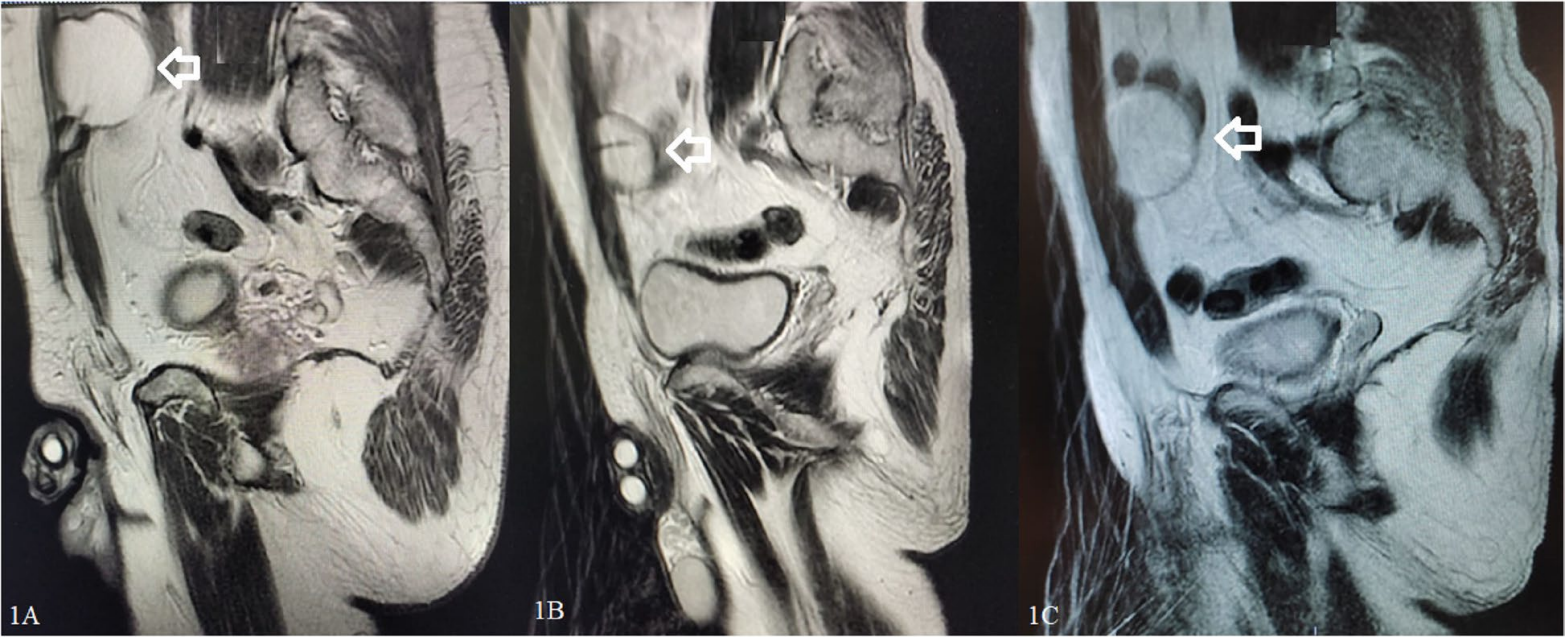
Abdominal magnetic resonance imaging of different patients at postoperative 2nd year (1A), 4th year (1B) and 6th year (1C). The arrows shows the location of the inflatable penile prosthesis reservoir in all patients
